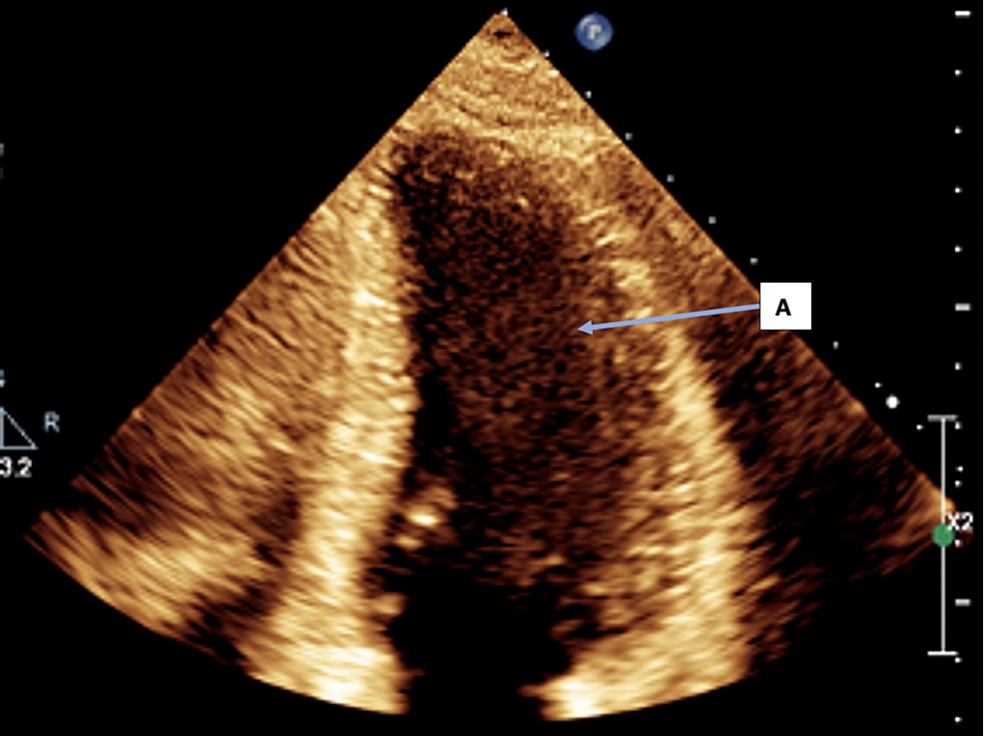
Post-treatment TTE: Apical 4 chamber (A4C) view in diastole showing preserved LV systolic function. A: The left ventricle appears of normal size in diastole in this still image.
